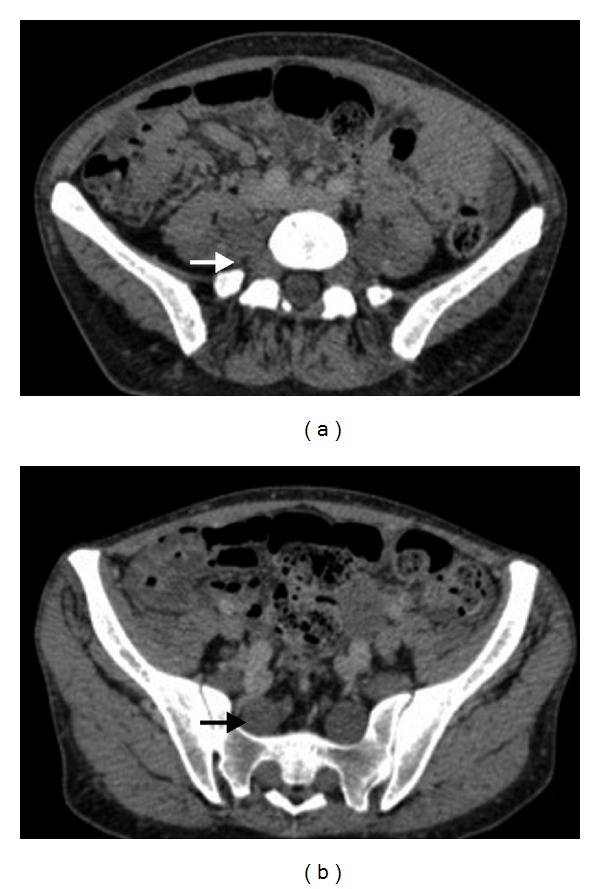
A 34-year-old woman with neurofibromatosis type I. Axial CT show plexiform neurofibromas of lumbar and sacral nerve roots.
